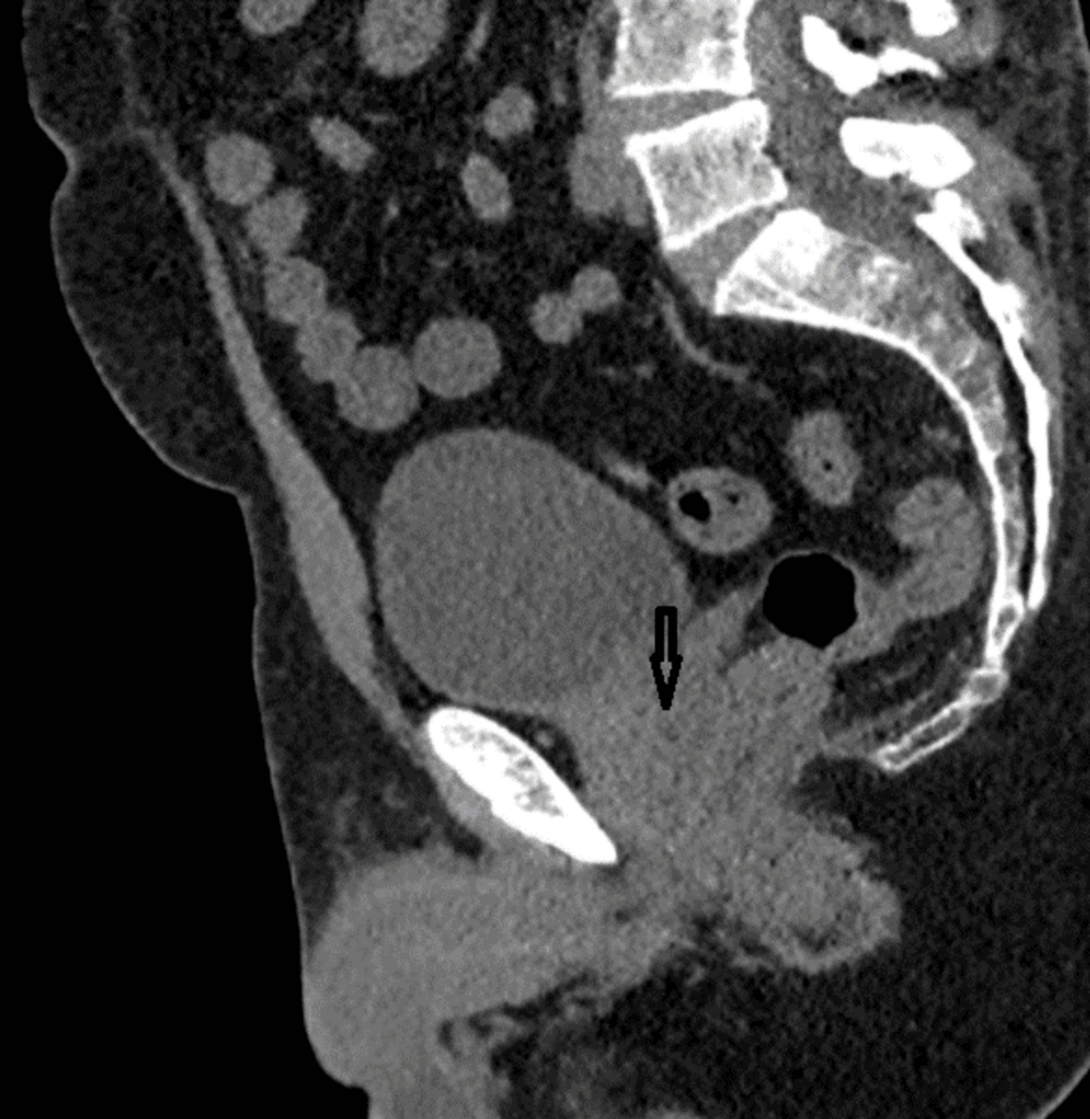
Post-procedure low-dose CT (sagittal) showing clearance of the calcification at the same level (arrow points to the location of previous calcifications), confirming an unobstructed beam path (original image). Source: Authors; no copyrighted material used; permission not required.
